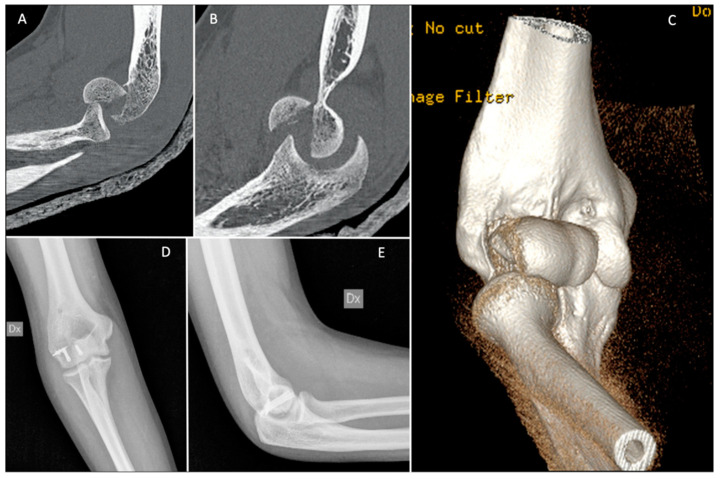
(A–C) Pre-operative CT scan; and (D,E) post-operative X-rays of a Dubberley 2A fracture fixed with independent antigrade headless screws.
